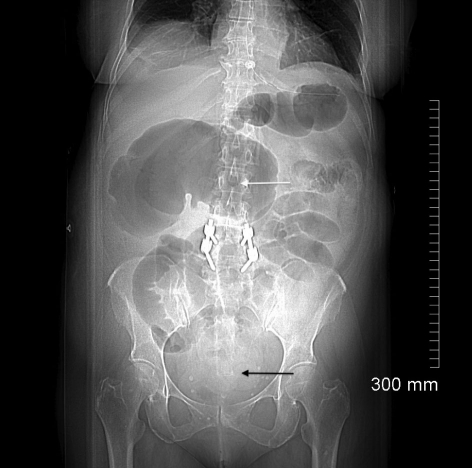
CT scout image demonstrates a dilated cecum in the right upper quadrant producing the classic ”coffee bean“ sign (white arrow). There are multiple dilated loops of small bowel present centrally within the abdomen with a paucity of gas in the distal colon (black arrow) suggestive of a complete intestinal obstruction. This patient had spinal instrumentation consistent with her previous history of spine surgery.
